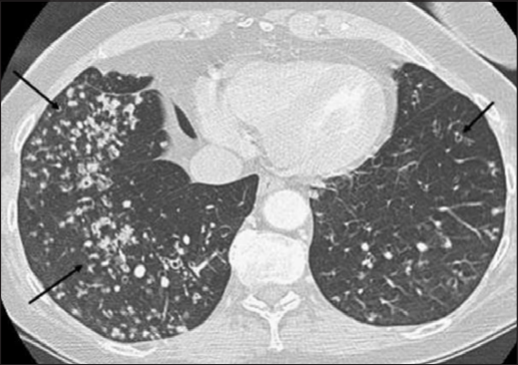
Miliary tuberculosis.* A 65-year-old female with dyspnea was admitted as her pneumonia was not improving despite treatment. The CT was performed with a suspicion of pulmonary embolism. The images show multiple small cavitary nodules in the upper and lower lobes on both sides. Microbiology proved positive for tuberculosis.
